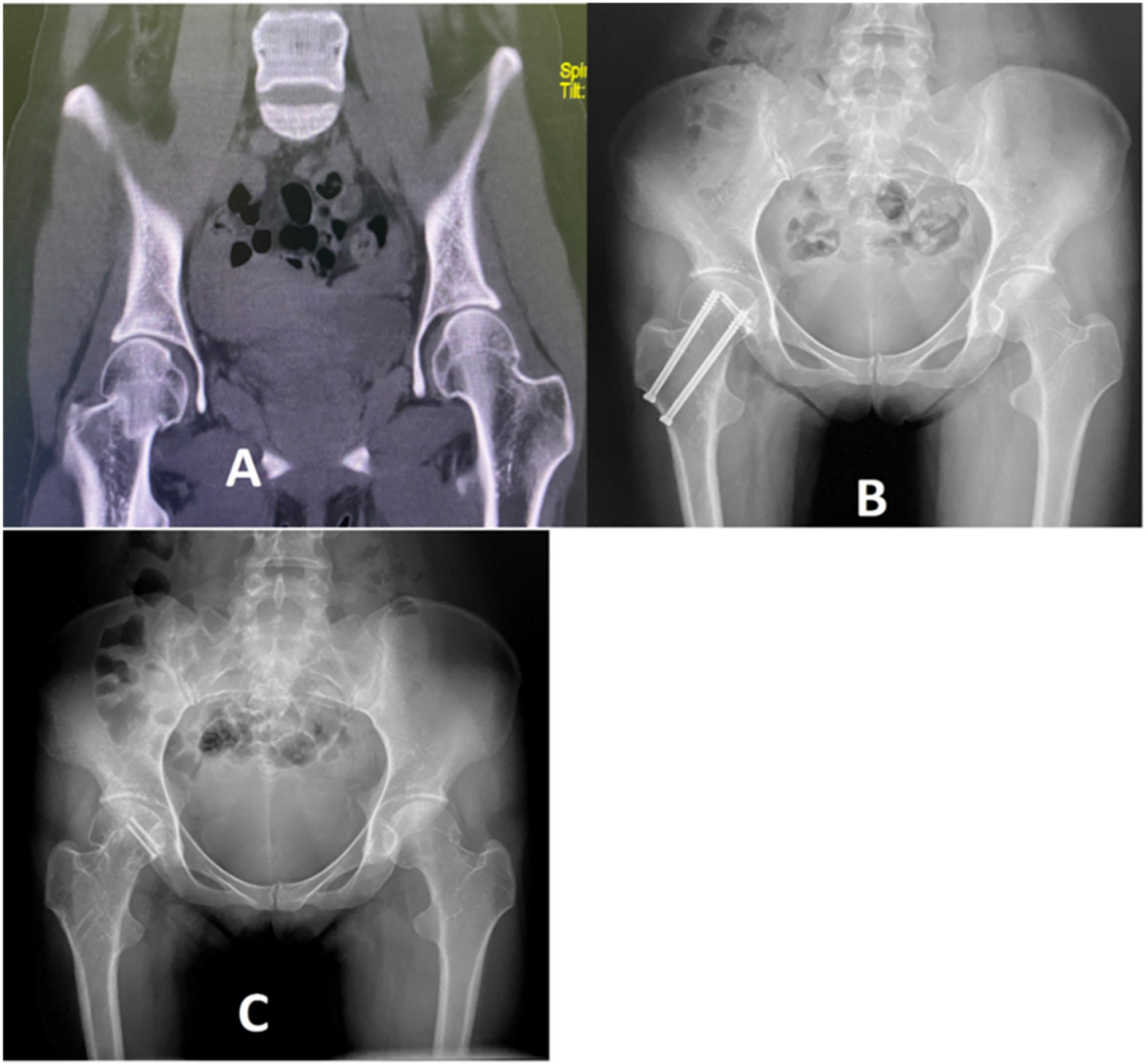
A 28y years old female who sustained a low energy fall at the workplace. (A) The pre-operative coronal view of CT scan revealed a right-sided femoral head and neck fracture. (B) The post-operative plain radiograph shows the internal fixation on the right femoral head and neck with two Herbert screws and three cannulated screws. (C) An x-ray taken four years later displays the removal of the cannulated screws from the right hip, with the Herbert screws still in place on the femoral head with no indications of avascular necrosis or post-traumatic arthritis.
